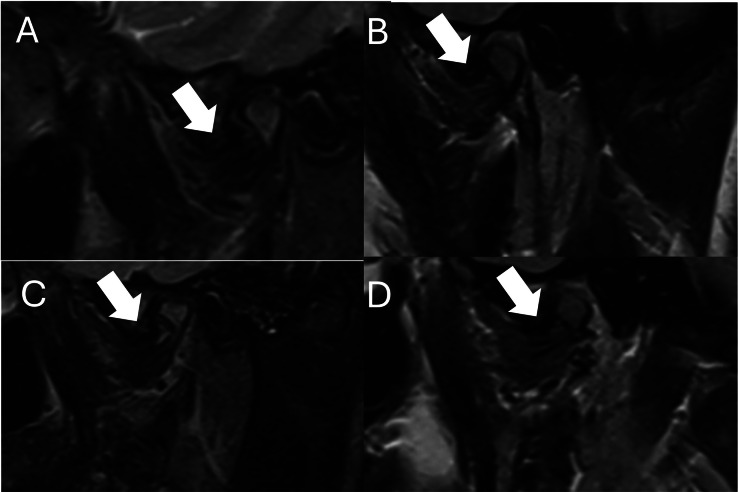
MRI T2 Weighted Imaged. Sagittal view. Arrows indicate disk displacement (A) Right side close mouth. (B) Right side open mouth. (C) Left side close mouth and (D) Left side open mouth.
